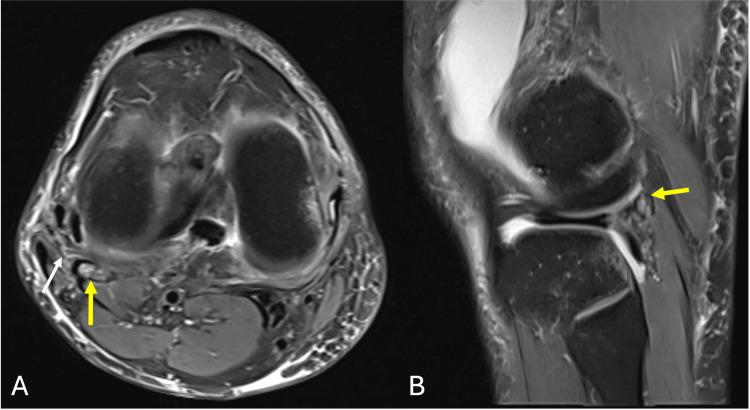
PDFS MRI (A) axial PDFS and (B) sagittal images of the right knee demonstrating bone marrow edema within the fabella, seen embedded within the lateral gastrocnemius tendon, with a visible fracture line (yellow arrows) consistent with a fabella fracture. Mild injury to the secondary stabilizers of posterolateral corner structures are also noted as shown by white arrow PDFS : proton density fat-saturated
